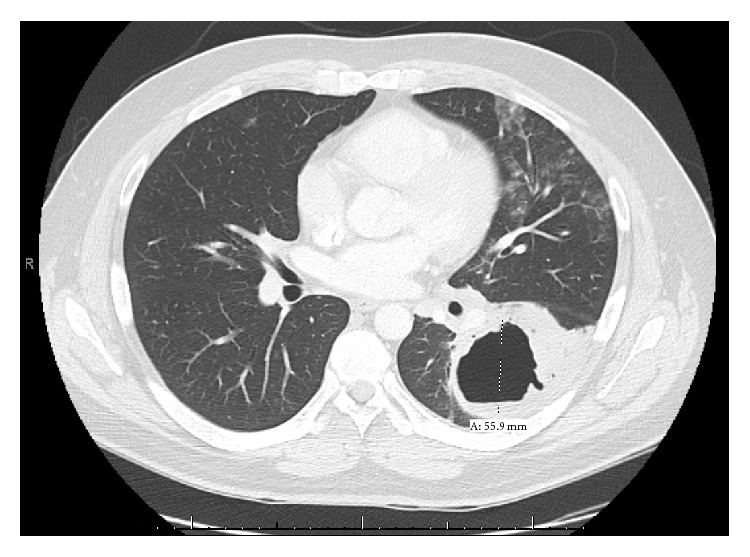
Chest computed tomography of left lower lobe superior segment cavitary lesion with air fluid level, largest diameter 5.59 cm.
